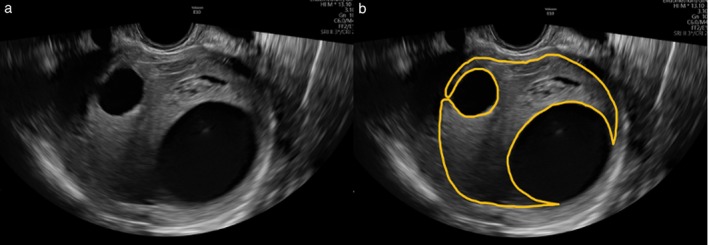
Grayscale ultrasound images of sarcoma without (a) and with (b) manually segmented tumor mask (yellow contour) obtained using Aliza Medical version 2.3.10 software. Only solid tissue was included in region of interest; cystic areas larger than 5 mm in maximum diameter were excluded.
